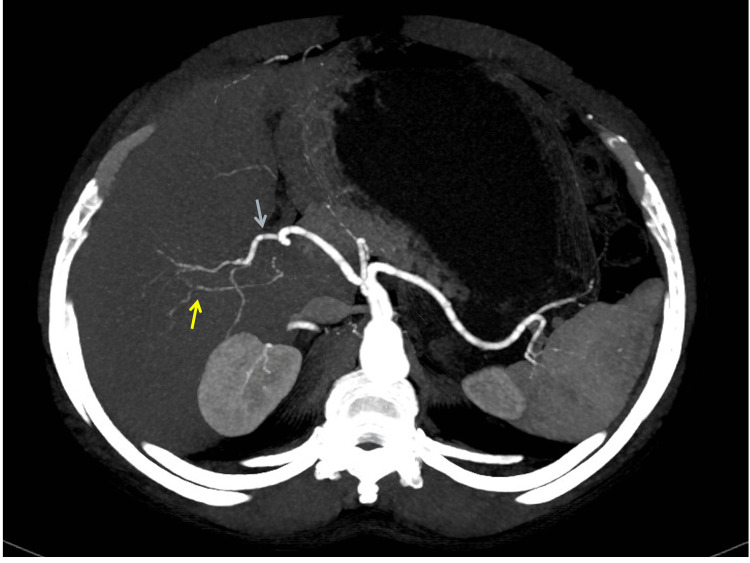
Contrast-enhanced CT of the abdomen shows segments 5, 7, and 8 arteries (yellow arrow) arising from the right hepatic artery (blue arrow).
